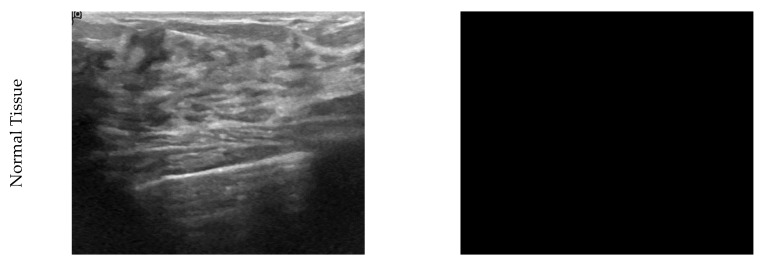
Ultrasound and clustered images of breast cancer for benign, malignant, and normal tissue conditions.
